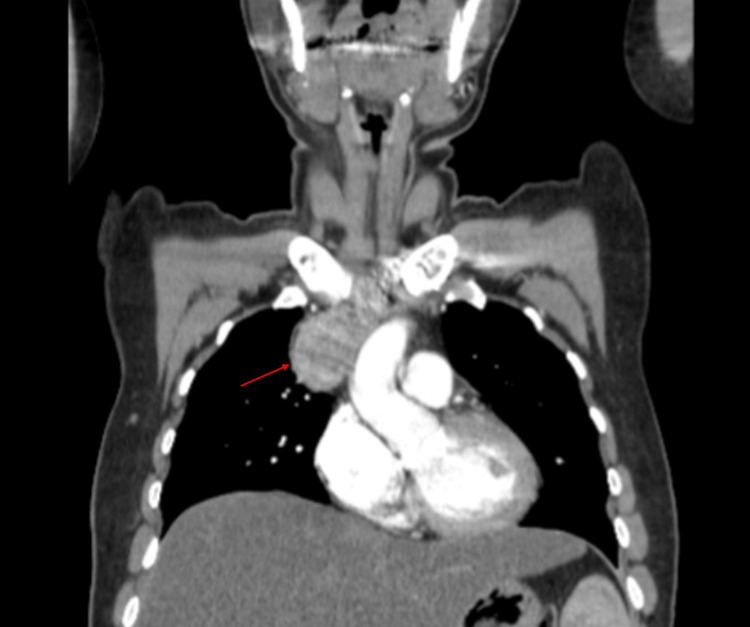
Contrast-enhanced computed tomography, coronal view, showing a mass in the superior mediastinum measuring approximately 5 x 8 cm, with no enhancement after contrast administration.
